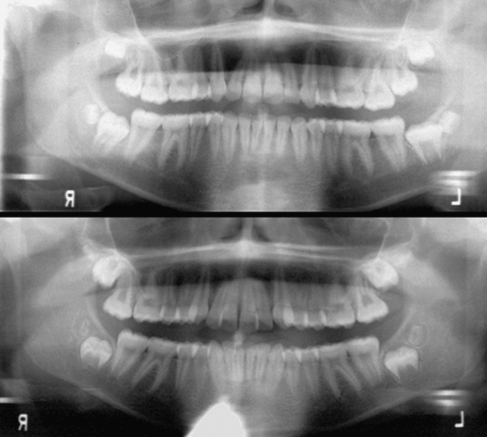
Panoramic radiographs of two American Black adolescents with a developing fourth molar in each quadrant. These supernumerary teeth are reduced in size, but exhibit differentiation into multiple occlusal cusps. These extensions of the molar fields could conceivably involve differences in these subjects’ ectodysplasin, as described by Tucker and Sharpe.^31^
